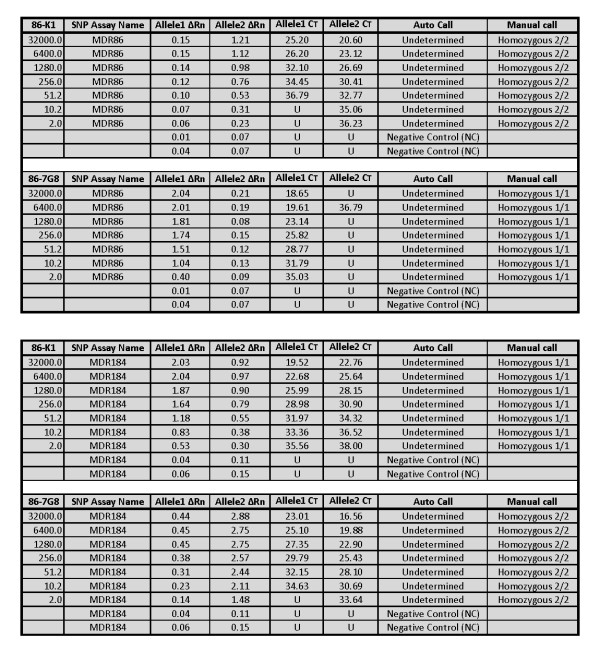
Manually called SNP assays. Data showing the manual call of MDR86 and MDR184 SNP assays. The automatic call for these SNP assays during these runs were undetermined however as the data shows, Allele1 and Allele2 ΔRn in these SNP assays were clearly separated. This data shows manual calls can be made with great confidence regardless of whether automatic calls are made or not. In addition, CT values showed a clear and distinct difference between the two alleles.
